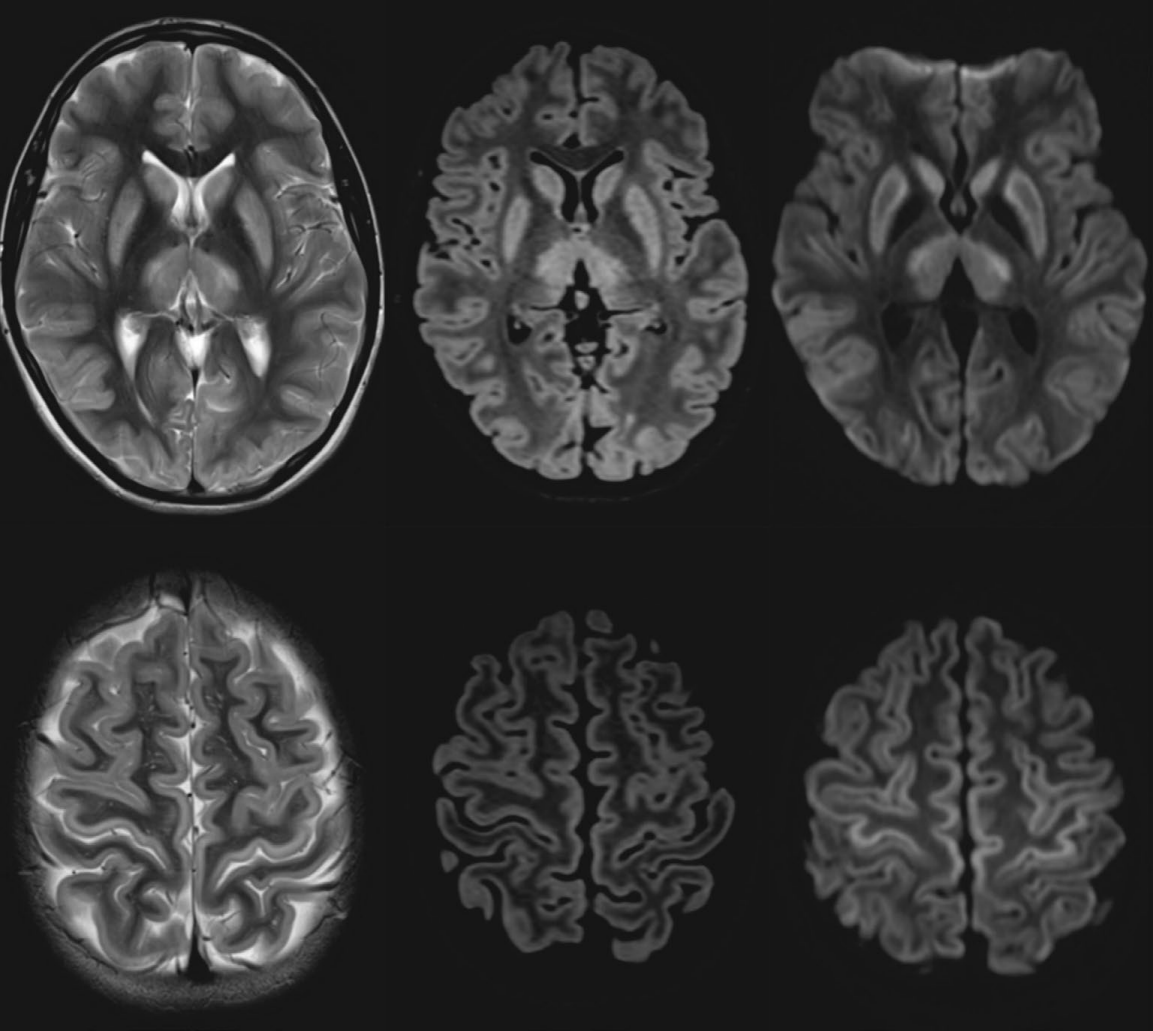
MRI with axial T2W, FLAIR and DWI images taken at the level of the basal ganglia (top row) and towards the vertex (bottom row) showing bilateral symmetrical basal ganglia, thalamic and cortical signal changes. The cortical changes are more evident within the precentral gyri (primary motor cortex). Subtle early subcortical white matter involvement is seen in the occipital lobes.
